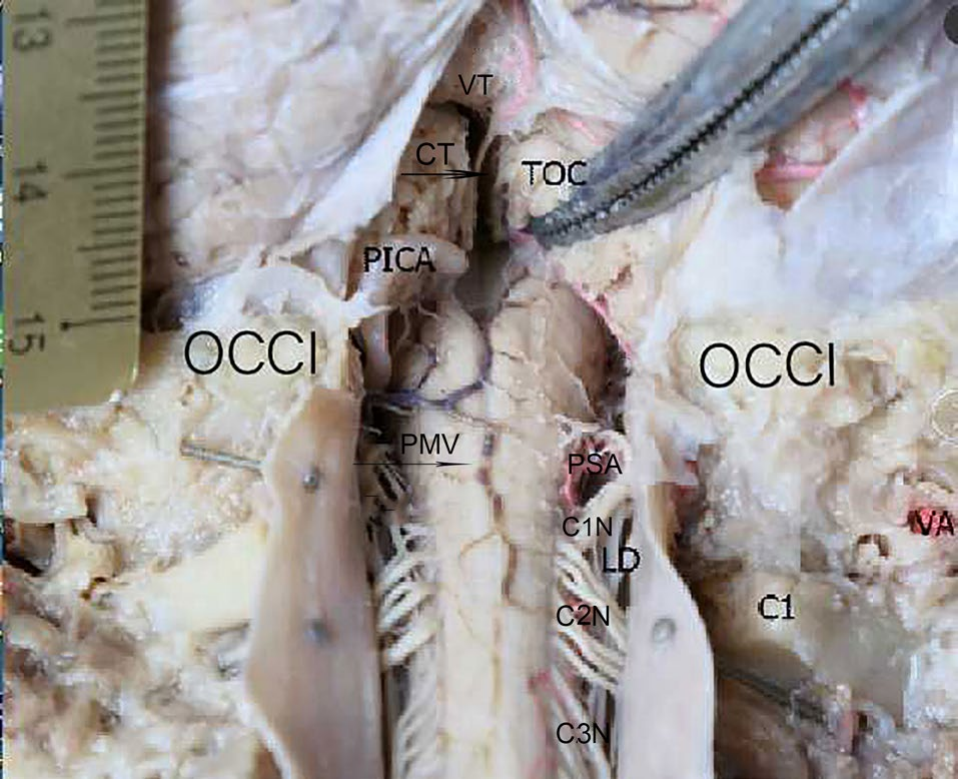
Posterior view of the cerebellum, medulla oblongata, and the upper cervical spine with the arachnoid mater having been removed. The enlarged subarachnoid space was exposed, and its was observed to be below the foramen magnum (C0) and dorsal to a series of structures consisting of the C1-3 spinal segments, their spinal nerve roots, and the denticulate ligaments. VT, the tuber of the vermis; OCCI, occipital bone and the foramen magnum; C1, the atlas; LD, the denticulate ligament of the spinal cord; PICA, the posterior inferior cerebellar artery; VA, the vertebral artery; TOC, the tonsil of the cerebellum; CT, the choroid tissue of fourth ventricle; PSA, the posterior spinal artery; PMV, the posterior median vein of the spinal cord; C1N, the root of the first cervical spinal nerve; C2N, the root of the second cervical spinal nerve; C3N, the root of the third cervical spinal nerve.
